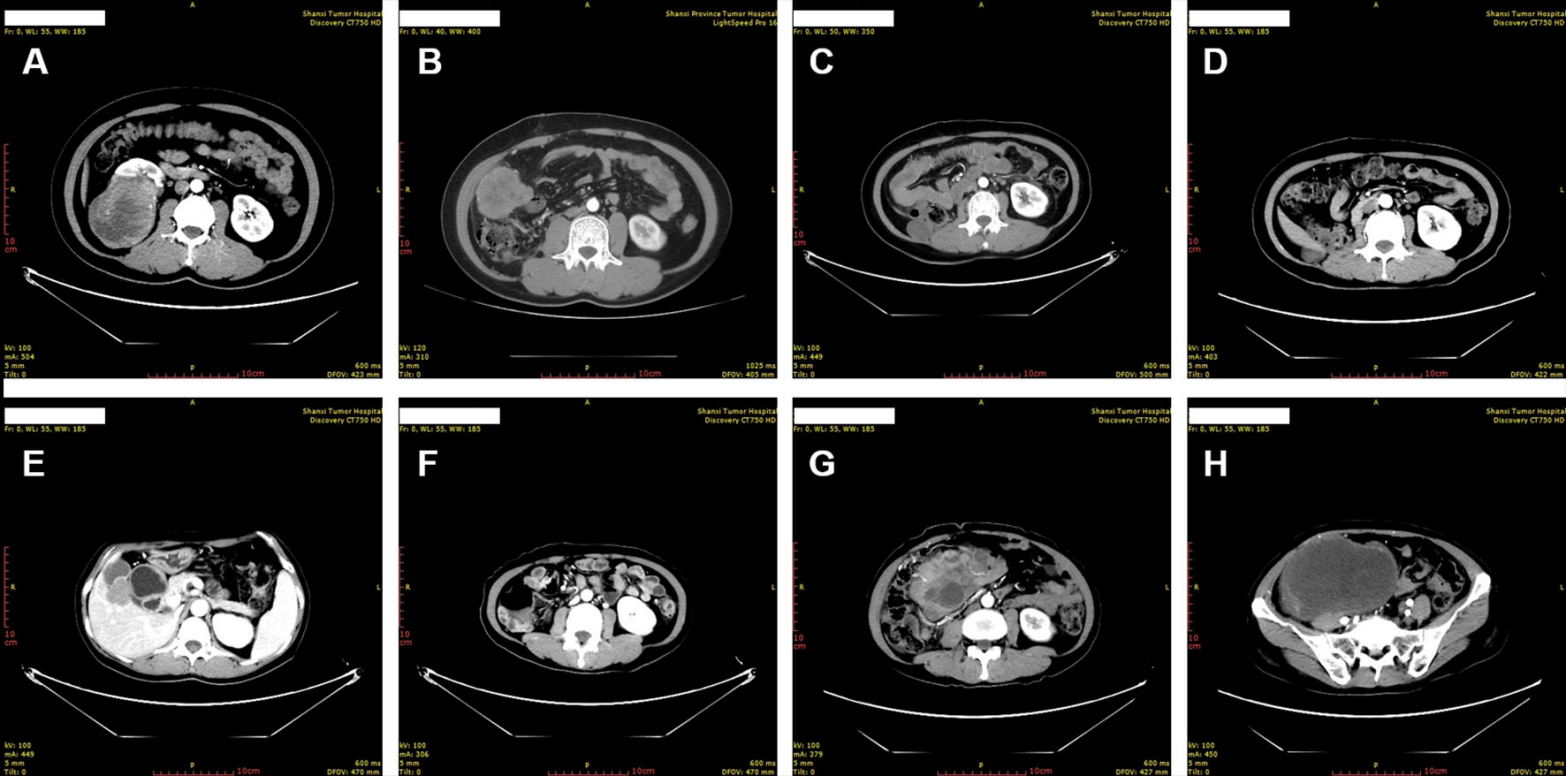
Chronological computed tomography of the perivascular epithelioid cell tumor. (A) The primary tumor was identified as right kidney mass. (B) Metastases were identified in the right abdominal cavity 10 months after resection of the primary tumor. (C, D) Shrinkage of the abdominal cavity tumor was identified 3 months (C) and 6 months (D) after the initiation of anti-VEGFR TKI treatment, respectively. (E, F) Continuous shrinkage of the abdominal cavity tumor and appearance of new metastases in gallbladder fossa was identified 15 months after the initiation of anti-VEGFR TKI treatment. (G, H) New, widespread metastases were identified in the abdomen and pelvis 15 months after the initiation of the MTOR inhibitor everolimus treatment.
